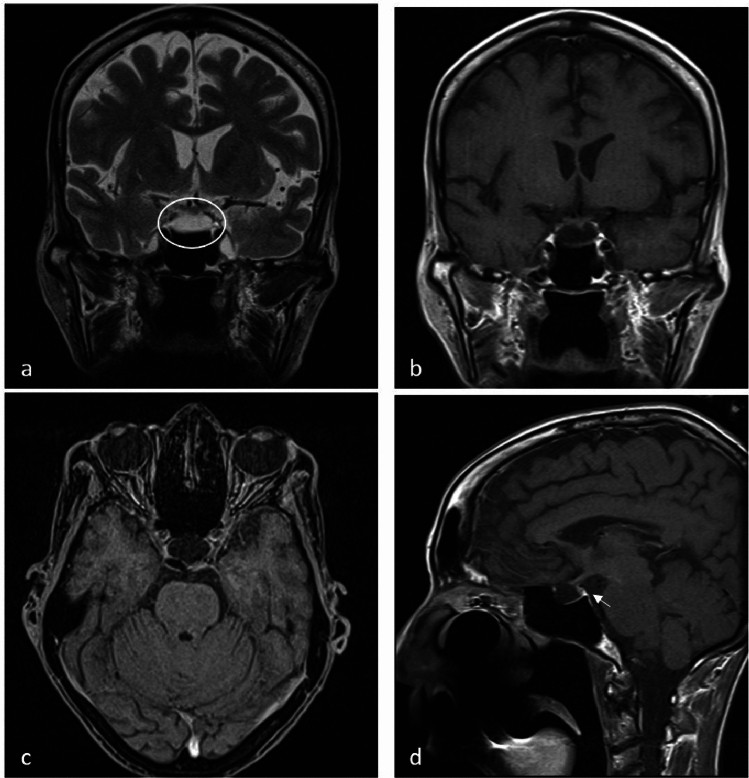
Encephalic MRI with contrast enhancement from which a picture of partial empty sella (circle) emerges with thinned and displaced parenchyma of the pituitary gland on the sella floor. A normal hyperintense neurohypophysis with the pituitary peduncle (arrow) regularly contrast enhanced, pushed back and deviated to the left. a: T2 sequence, coronal; b: T1 sequence with CE, coronal; c: T1 sequence with CE, axial; d: T1 sequence with CE, sagittal
